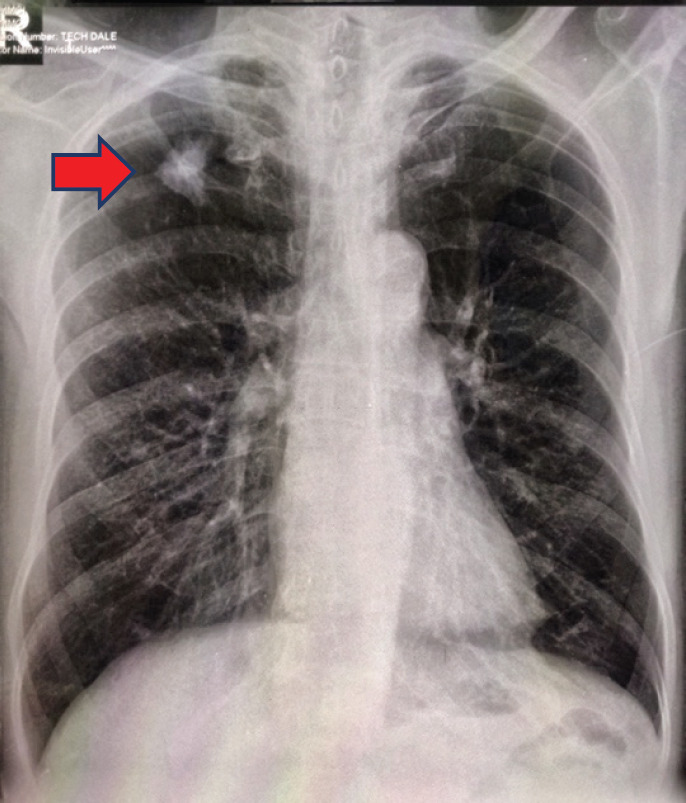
Chest radiography showing ill-defined infiltrates in the right upper lobe (red arrow).
